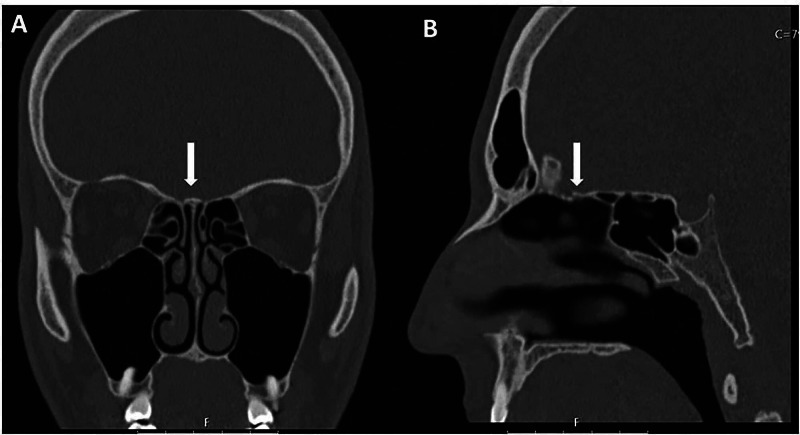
Cerebral and Paranasal Sinuses CT scan The CT scan did not reveal any anatomical anomalies of the cranium or facial massif. The nose and peri-nasal sinuses showed normal configuration and transparency, with no evidence of obstructive lesions. The region corresponding to the olfactory bulbs (arrow) did not show changes in density suggestive of expansive lesions or fracture lines (A - coronal cut; B - axial cut)
